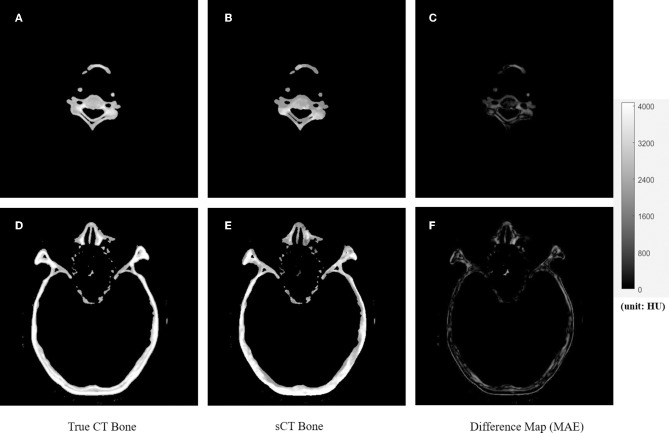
The comparison of true CT and synthetic CT for bone. The first column is true CT images. The second column is the synthetic CT images. The third column is the difference maps. (A–C) Showed bone in the neck region. (D–F) Showed bone in the head and nasal region. Gray bar indicated the mapping from CT number to gray scale in the difference maps.
